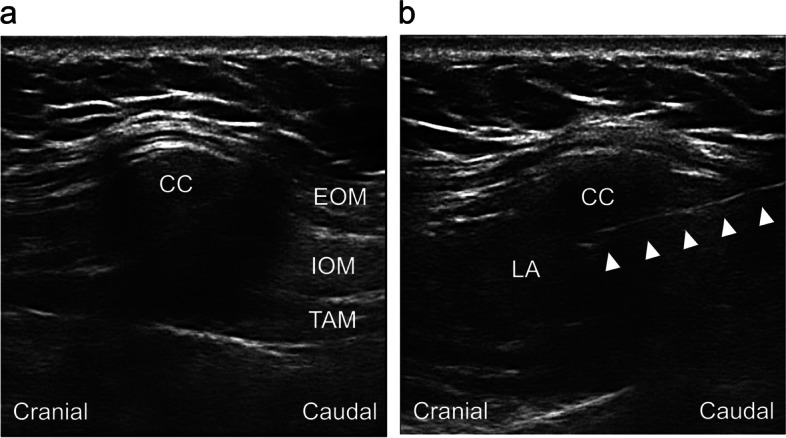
Ultrasound image of M-TAPA. a An appropriate image for M-TAPA. b Image obtained after injecting the local anesthetic solution. The arrows denote the Touhy needle. *M-TAPA* modified thoracoabdominal nerve block through perichondrial approach, *EOM* external oblique muscle, *IOM* internal oblique muscle, *TAM* transversus abdominis muscle, *CC* costal cartilage, *LA* local anesthetic
